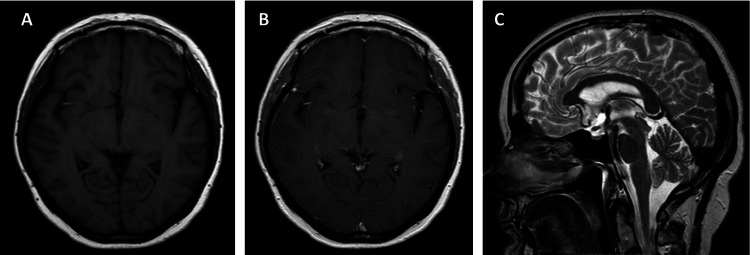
Postoperative MRI - T1 and T1-weighted with no enhancement and no residual tumor. T2 with no recurrence of the tumor and no hydrocephalus.
